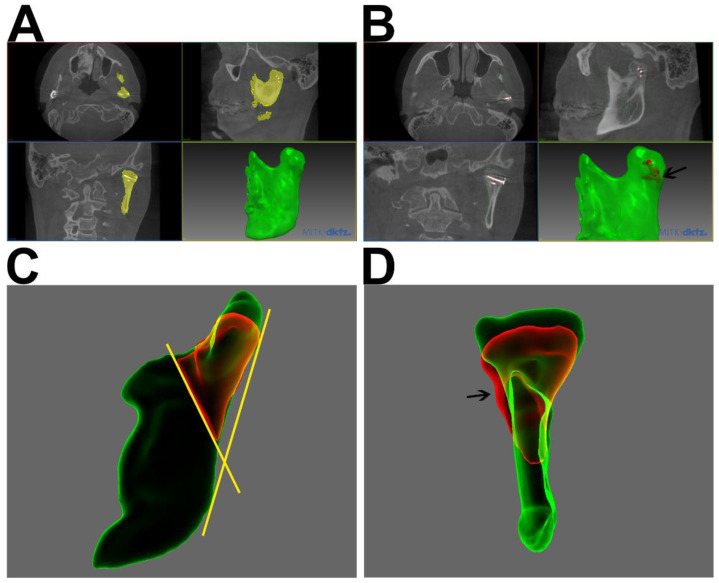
(A) Follow-up CBCT-scan of a 21-year-old male patient with a bilateral fracture of the condylar head. Segmentation of the left mandibular ramus in MITK Workbench ^®^ (yellow) and the generated smoothed STL-file (green) are shown. (B) Same patient as (A) with segmented and visualized osteosynthesis material (black arrow, three positioning screws). (C) Lateral view on the superimposition of segmentations of CBCT-scans at T1 (green) and T2 (red) (Artec Studio^®^); condylar neck of T2 (red) already split at the defined line (yellow) between most inferior point of the sigmoid notch and the most posterior boundary of the mandibular foramen. (D) Dorsal view of (C); remodeling of the condylar head is clearly visible with loss of height. Especially at the lateral aspect callus and resorption at the screw head are visible (black arrow).
